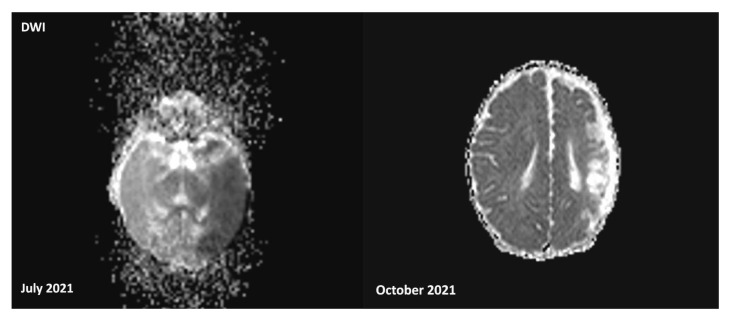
Brain MRI in DWI performed at birth, and after 4 months, showing both acute and subsequent notable sequelae from ischemic stroke in the territory of the left middle cerebral artery, moreover, revealing restricted areas in the left thalamus and left anterior pons, suggesting early Wallerian degeneration.
